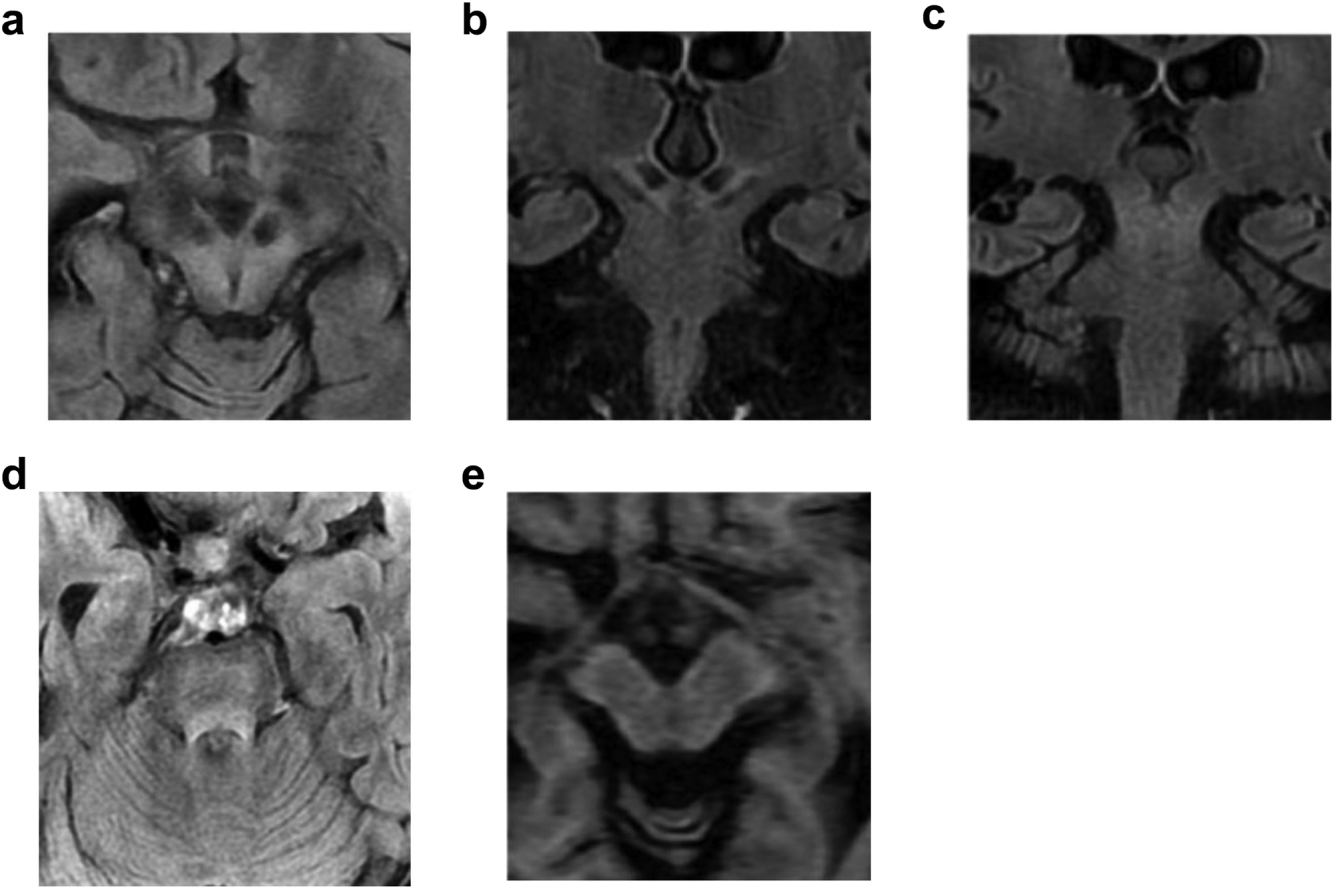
Conventional brain MRI of WD. Lesions were hyperintense in the midbrain and cerebral peduncle and hypointense in the substantial nigra and red nucleus on (a) axial and (b and c) coronal T2WI-FLAIR, (d) hyperintense in the pons on T2WI-FLAIR, and (e) hypointensity in the midbrain on axial T1WI.
